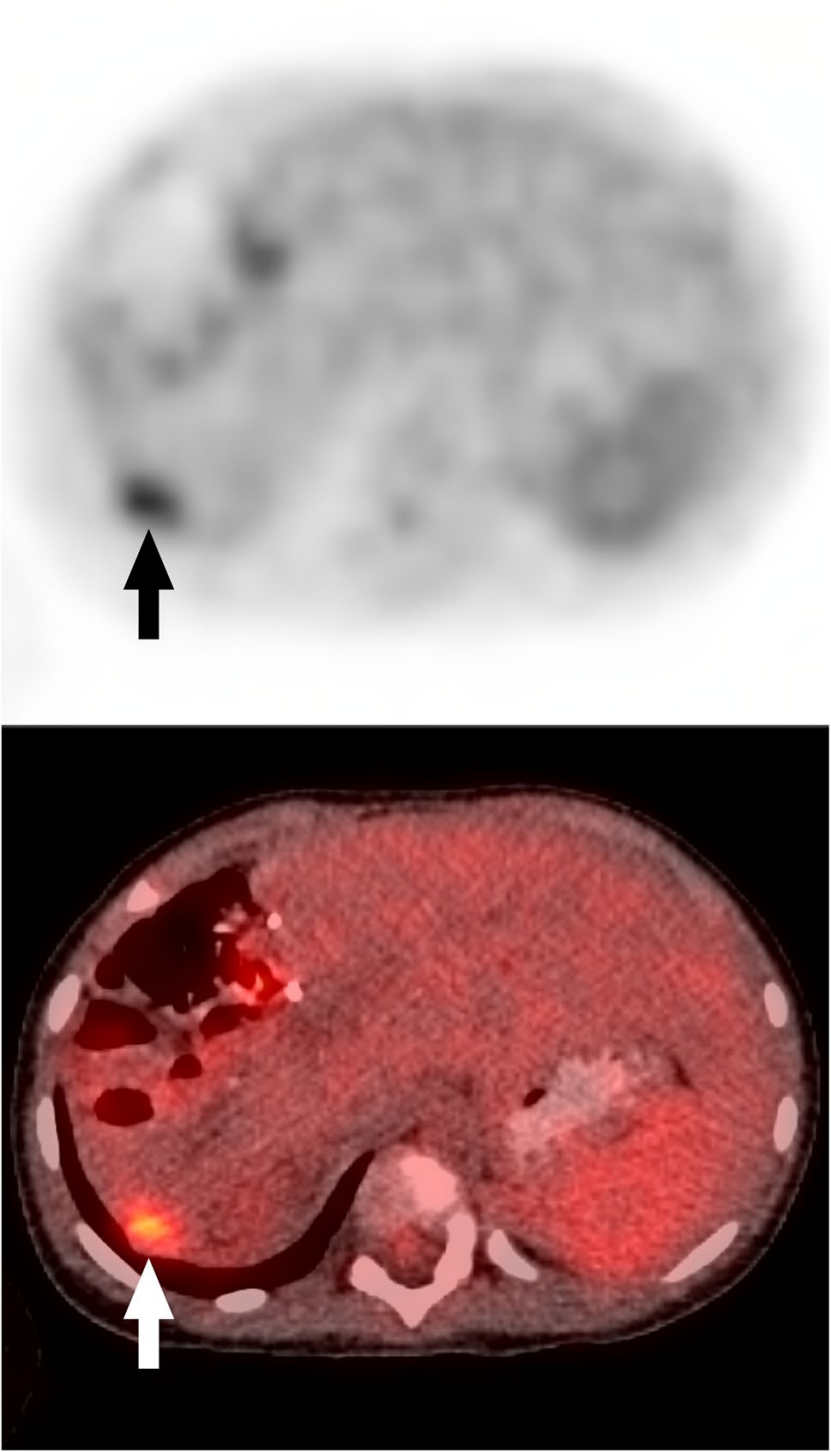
Axial PET and fused PET/CT images from ^18^F-FDG PET/CT in a 2-year-old girl with history of hepatoblastoma status post chemotherapy and partial hepatectomy with rising alpha fetoprotein. The primary interpretation reported “no evidence of FDG-avid recurrent or metastatic disease.” The secondary interpretation identified “focal abnormal FDG uptake in segment 7 of the liver compatible with recurrent/metastatic disease in context of rising AFP.” *Arrows* indicate the finding
